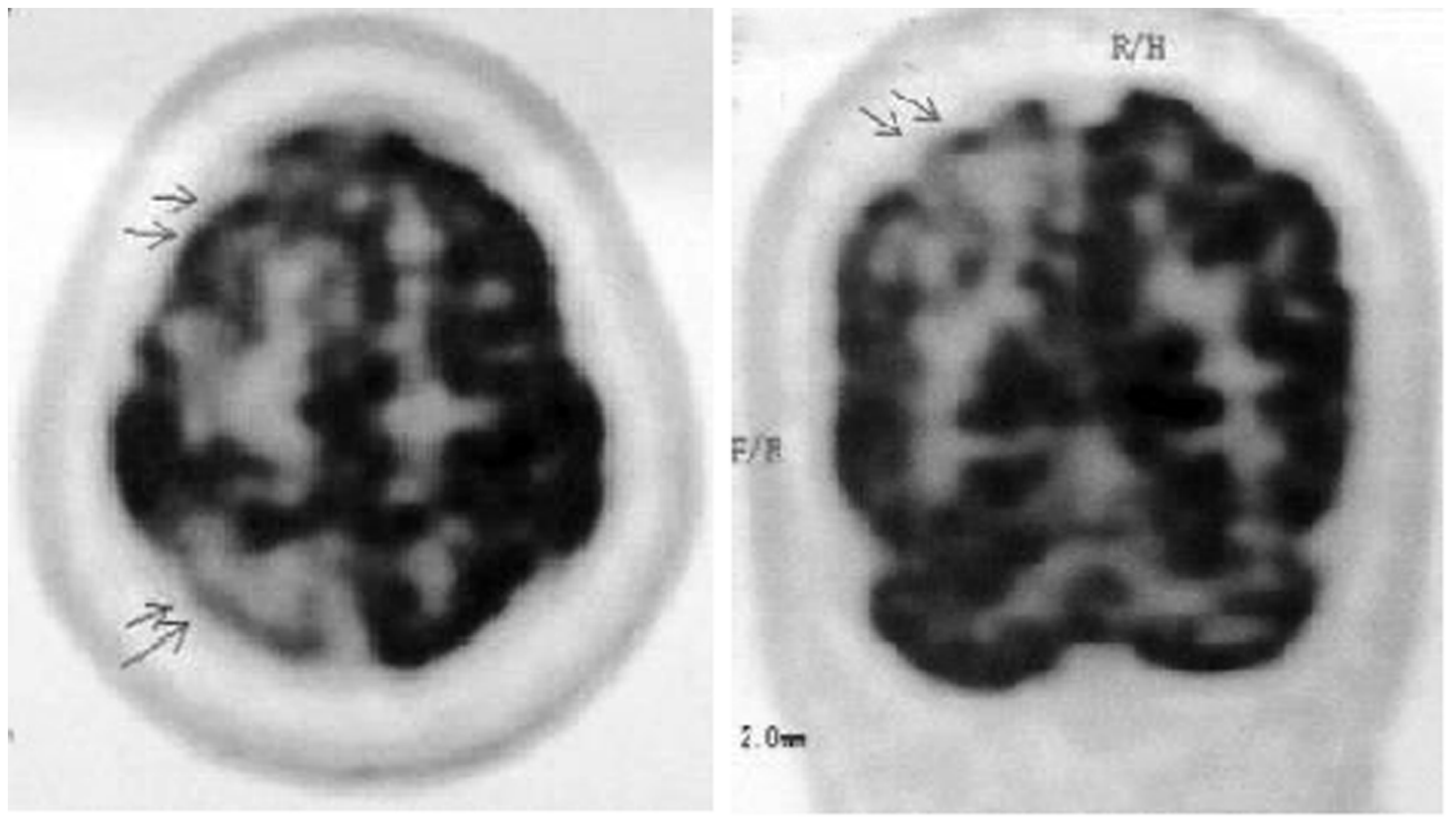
PET images of the same sCJD patient. The two images of positron emission tomography/CT scan in the same patient shows decreased ﬂuoro-2-deoxy-glucose (FDG) uptake in right frontal and parietal cortices.
